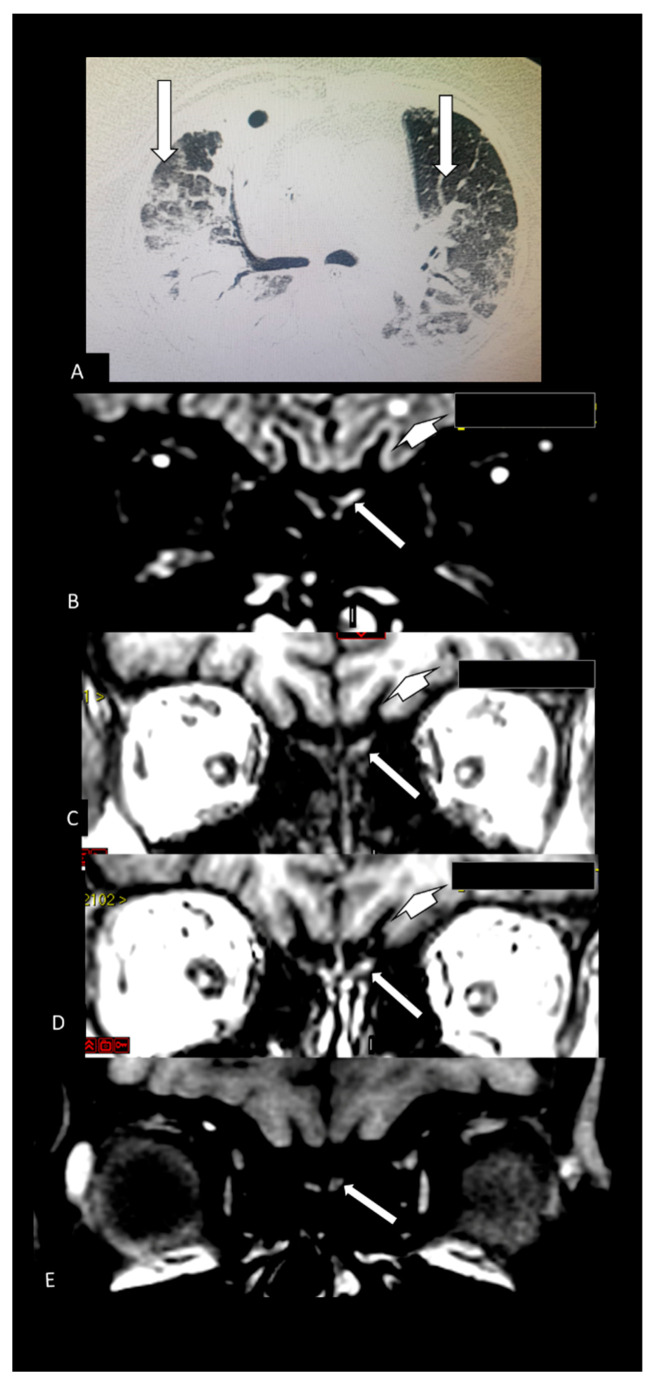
Case 27—The Chest CT shows more than 50% of typical lung opacity of COVID 19 pulmonary injury, bilaterally (A, arrows). The MRI shows hyperintense lesion on 3D FLAIR (B, arrow) [16] and also on pre (C, arrow) [16] and post contrast SPGR T1 WI (D, arrow) [16]. This is suggestive of component of probably methemoglobin in this left olfactory bulb lesion which seems be little bigger and asymmetric compared with the apparently normal right olfactory bulb. This asymmetry in size is better seen on FLAIR (B) [16]. There is also a small round hyperintense lesion in the subcortical white matter in the left frontal lobe on FLAIR (B, short arrow) which is hypointense on T1WI (C, short arrow) and does not enhance on post-contrast T1WI (D, short arrow) being nonspecific [16]. The MRI performed 3 months later shows on pre contrast fat suppression T1 WI (E) that there is reduction on hyperintensity of the left olfactory bulb lesion.
